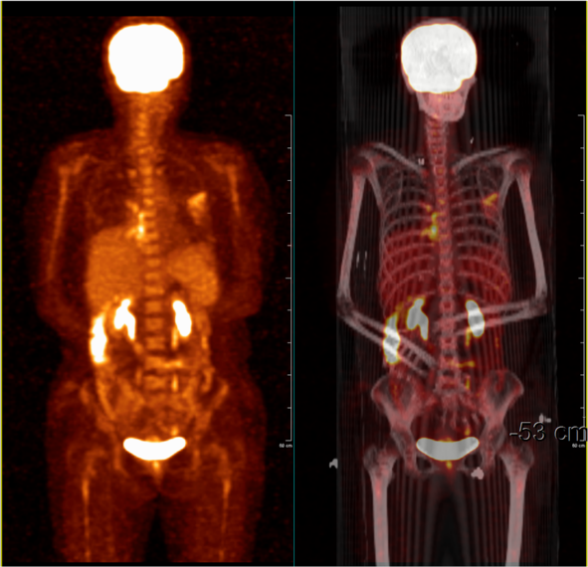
Whole body PET/CT after treatment showing resolution of high metabolic foci
